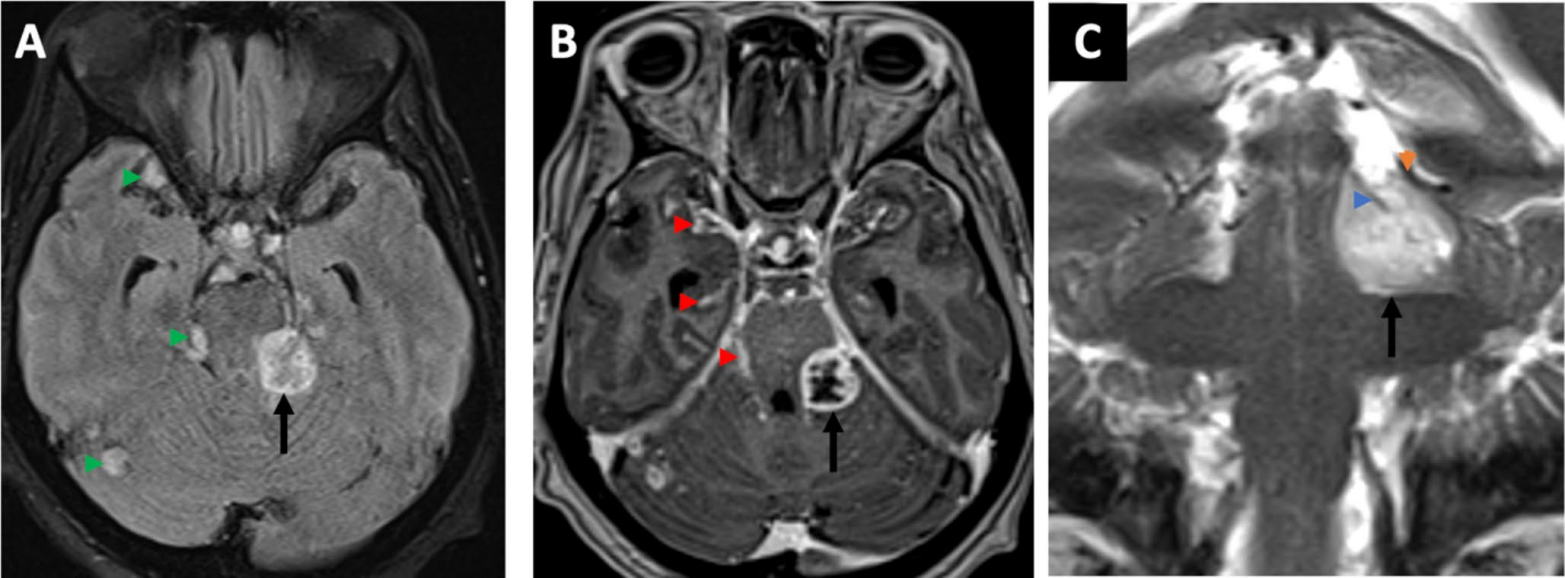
MRI brain. Axial FLAIR (A), Axial postcontrast T1-weighted MPRAGE (B), Coronal T2-weighted imaging (C). Nodular leptomeningeal tumor deposits are appreciable on FLAIR images as discrete foci of hyperintense signal abnormality within and around the basilar cisterns (green arrowheads in A). The largest tumor deposit (black arrow in A-C) demonstrated central necrosis on post-contrast imaging (black arrow in B) with corresponding hyperperfusion on cerebral blood volume map (not shown). More subtle diffusely disseminated smooth leptomeningeal enhancement is also noted on post-contrast imaging (red arrowheads in B). The extra-axial location of the largest tumor deposit compressing the left dorsal pons (black arrow in A-C) is better demonstrated on coronal T2-weighted images with lesional abutment of the tentorial leaflet (orange arrowhead in C) and encasement of the superior cerebellar artery flow void (blue arrowhead in C). *Abbreviations: MRI: magnetic resonance imaging*,* FLAIR: fluid-attenuated inversion recover*,* MPRAGE: Magnetization Prepared - RApid Gradient Echo*
